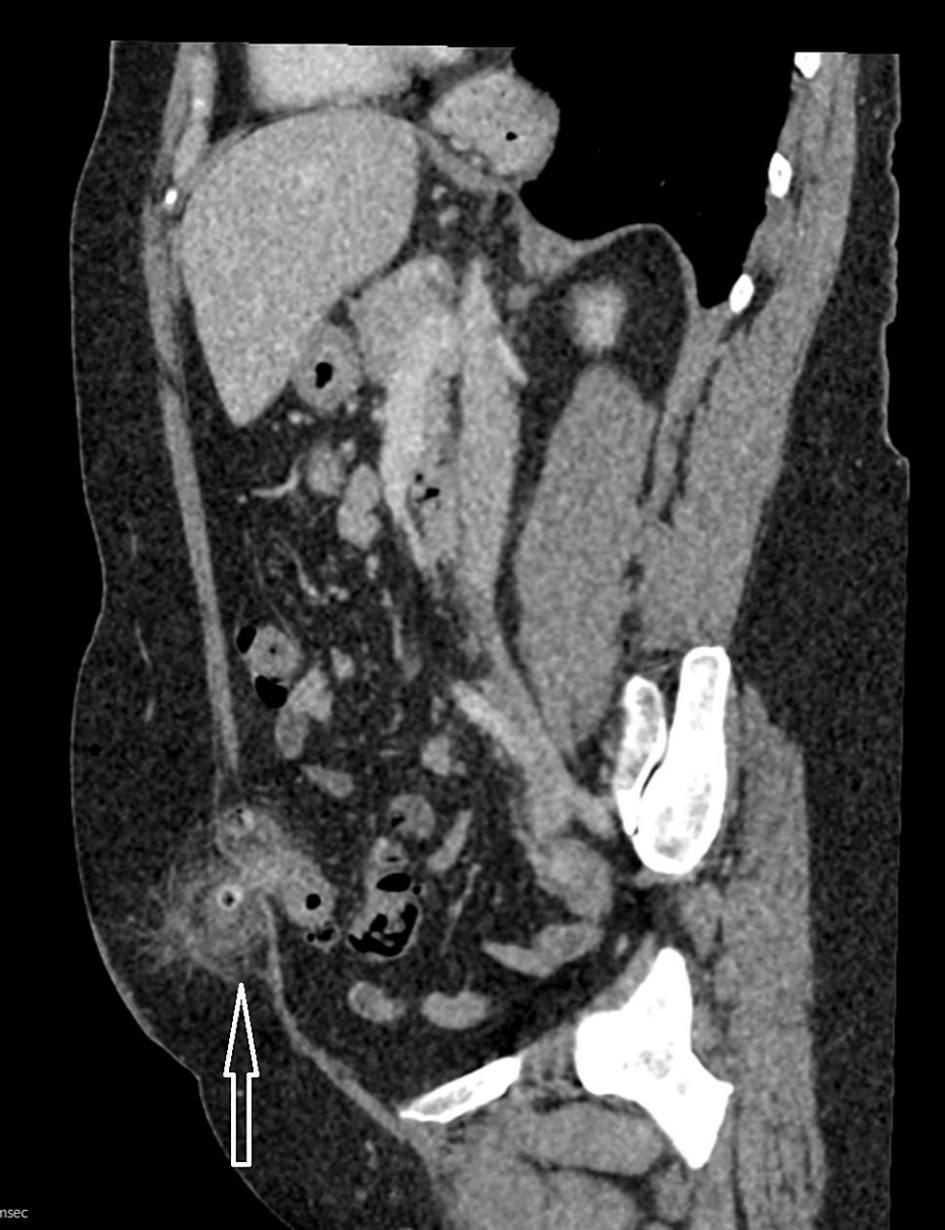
Sagittal view CT scan of the abdomen showing the ventral hernia containing sigmoid diverticulitis.
